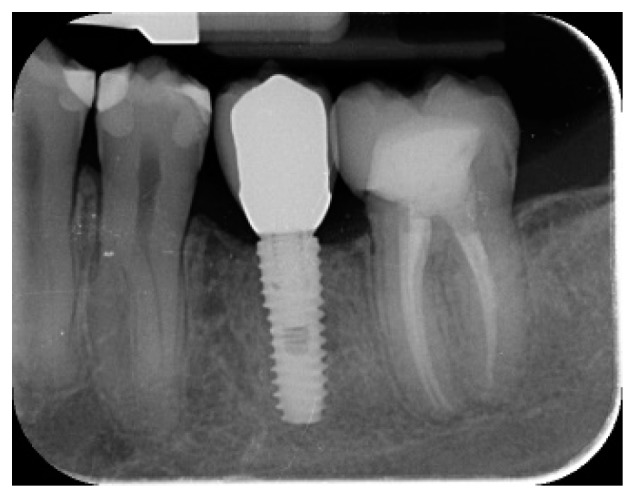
Radiographic view after prosthesis delivery.
